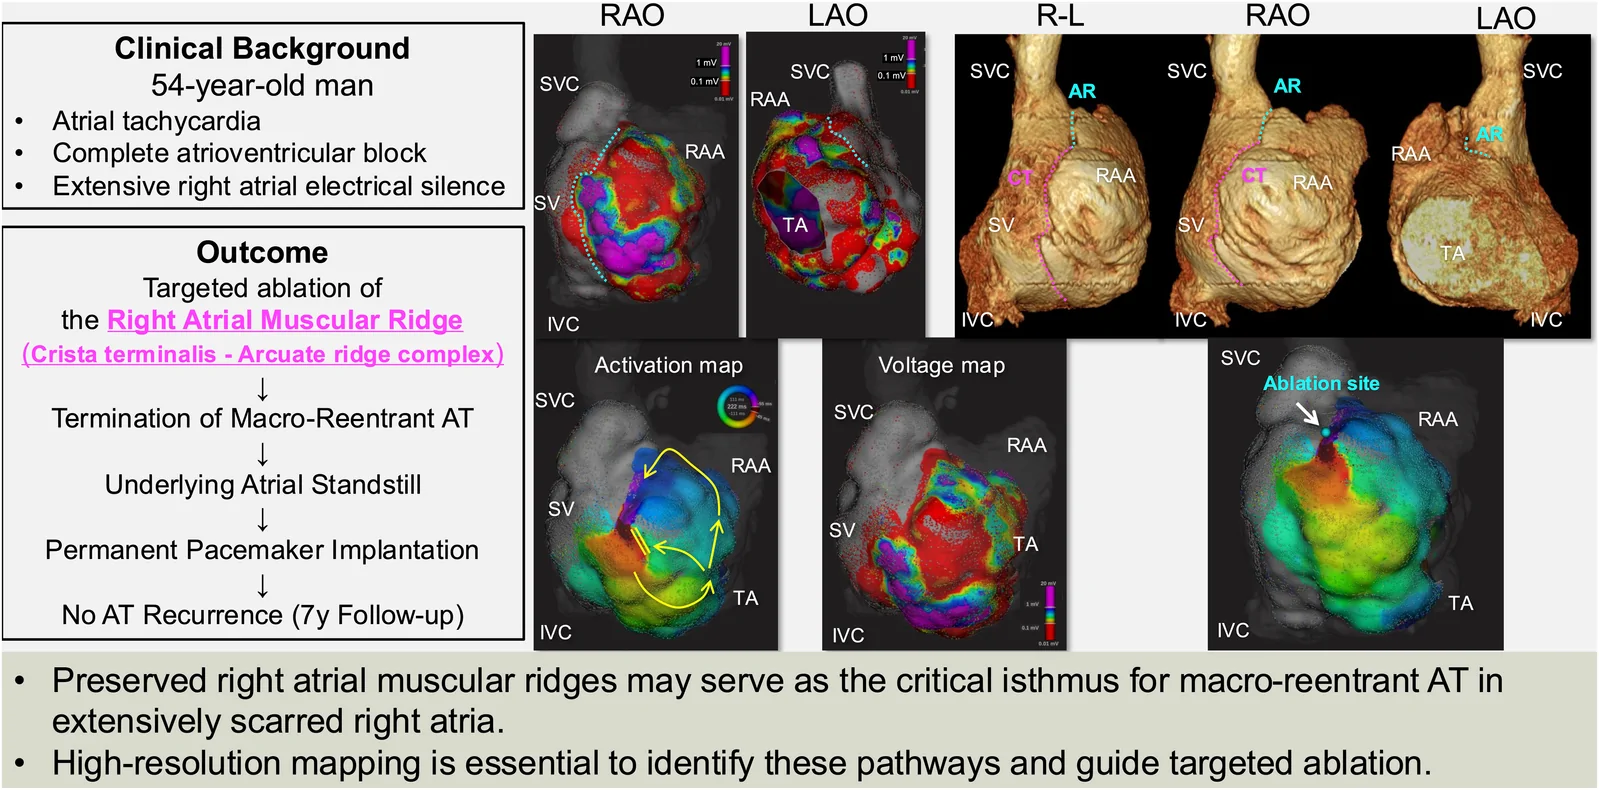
A Continuum of the Right Atrial Muscular Ridge, Including the Arcuate Ridge and the Crista Terminalis, Can Serve as a Critical Isthmus for Macro-Re-Entrant Atrial Tachycardia in a Patient With Partial Atrial Standstill Recognizing this infrequently reported structure and its anisotropic conduction properties is essential for electrophysiologists. AR = arcuate ridge; AT = atrial tachycardia; CT = crista terminalis; IVC = inferior vena cava; LAO = left anterior oblique; RAA = right atrial appendage; RAO = right anterior oblique; R-L = right-to-left view; SV = sinus venarum; SVC = superior vena cava; TA = tricuspid annulus.
